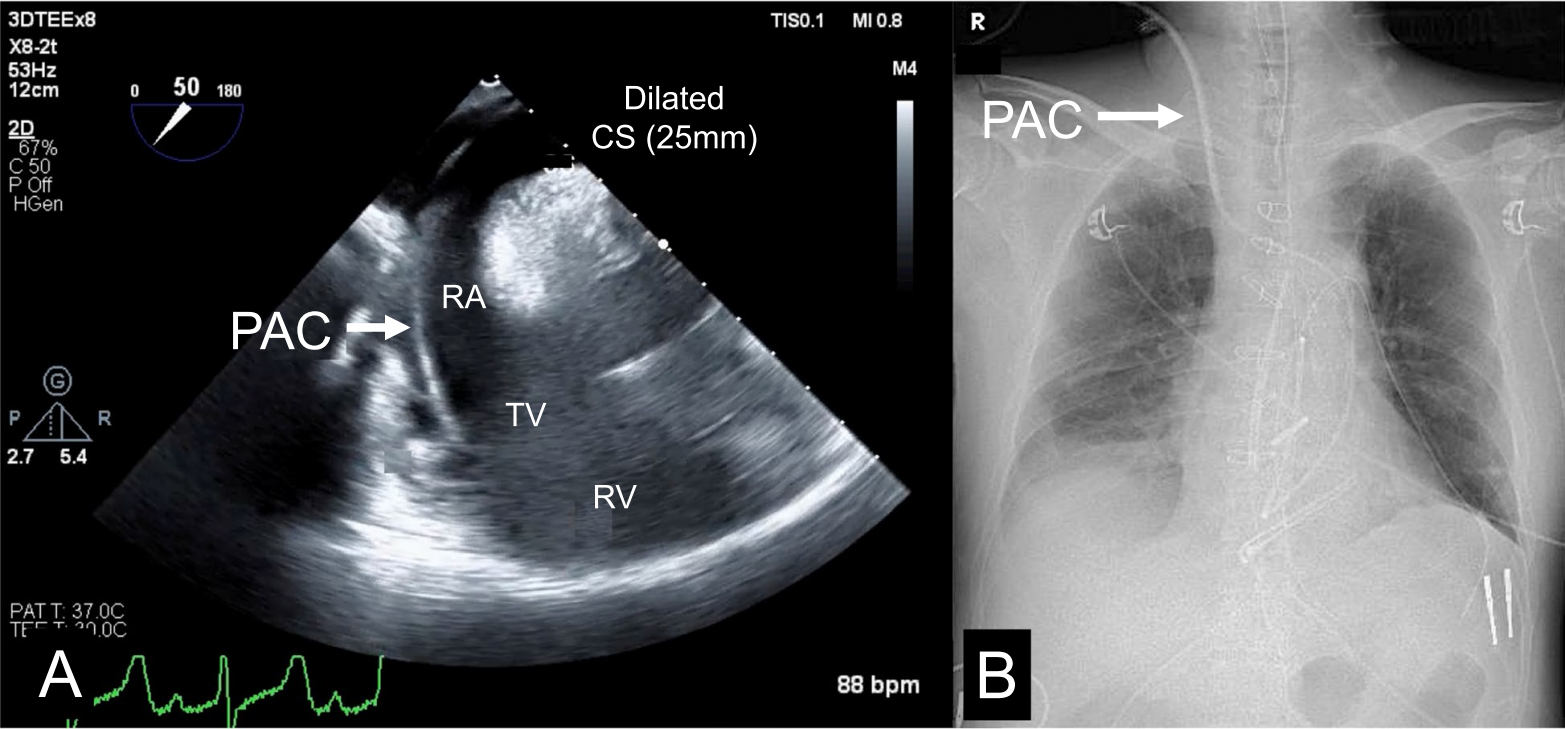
Intraoperative TEE findings and postoperative radiograph. A Transesophageal echocardiography image shows the mid-esophageal deep four-chamber coronary sinus view. The pulmonary artery catheter is placed through the coronary sinus and inserted into the right atrium, tricuspid valve, and right ventricle. B Postoperative thoracic radiograph taken in the operating room. CS: Coronary sinus. PAC: Pulmonary artery catheter. RA: Right atrium. RV: Right ventricle. TEE: Transesophageal echocardiography. TV: Tricuspid valve
